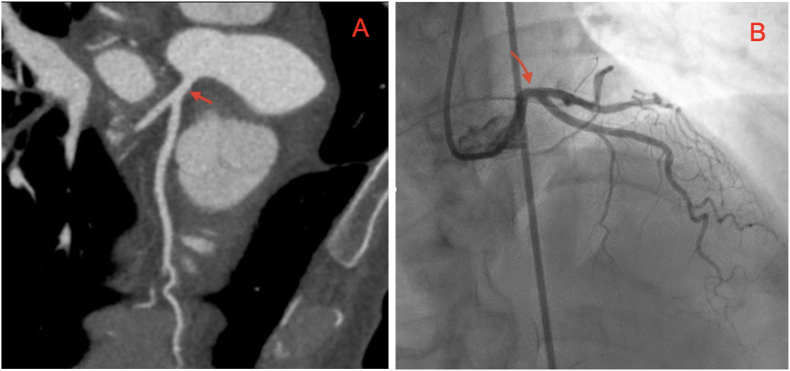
(A) Coronary Computed Tomography Angiography (CCTA) demonstrating the left coronary system with a patent left main coronary artery (red arrow). (B) Invasive Coronary angiography in the right anterior oblique cranial view showing normal left main coronary artery without stenosis (red arrow). (For interpretation of the references to colour in this figure legend, the reader is referred to the Web version of this article.)
